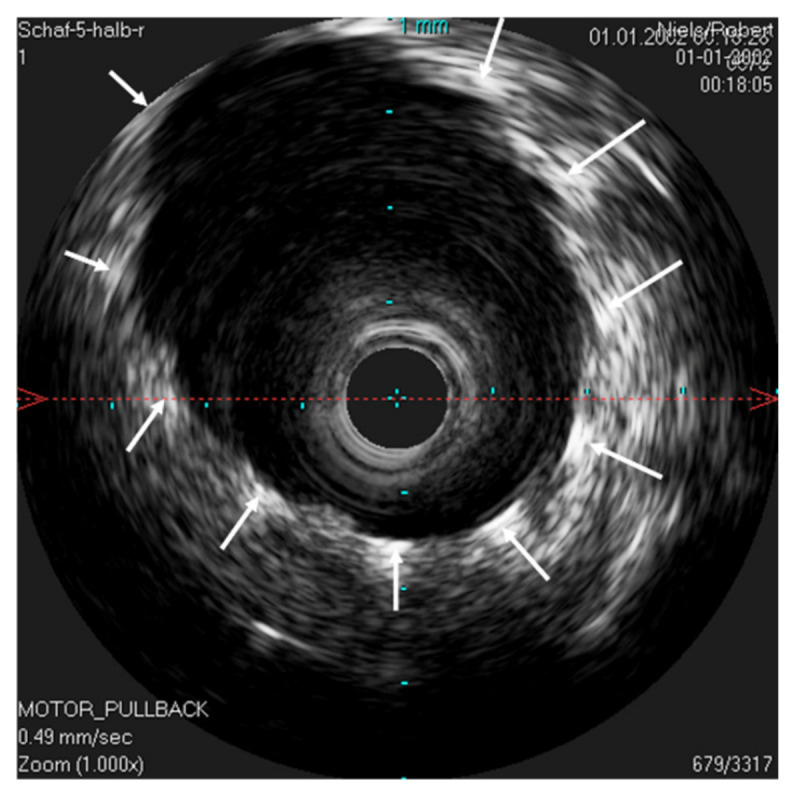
Ultrasound image of the stented ET. The lumen of the ET is opened by the unfolded stent. The IVUS probe is located in the lumen of the stent. The stent struts can be seen echogenically, and sonic extinction is marked with white arrows. Note: the ET was flooded with water before the scan to allow for sonic coupling.
